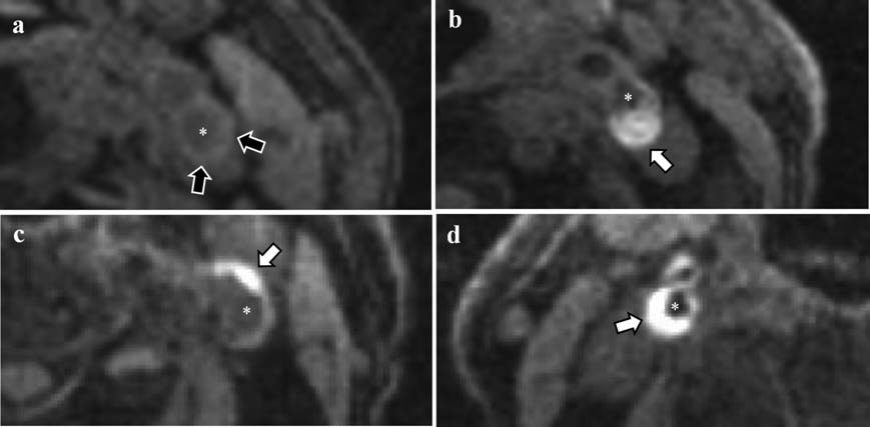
Axial views of T1-weighted water-selective magnetic resonance imaging to detect plaque hemorrhage of carotid arteries. Hyperintense signals (B–D, *white arrows*) reflect plaque hemorrhage in carotid arteries, black arrows (A) show absence of plaque hemorrhage, and asterisks indicate the lumen of internal carotid artery. (A) No signal hyperintensity. (B) Large moderately hyperintense plaque. (C) Small strongly hyperintense plaque. (D) Large strongly hyperintense plaque.
